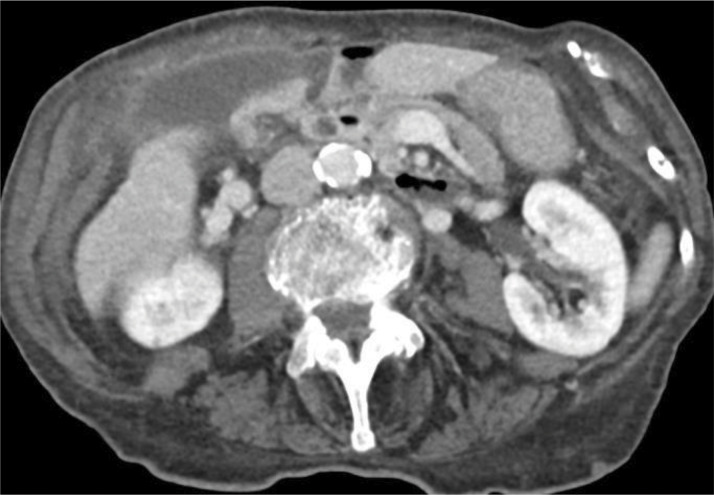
Axial image of a CT scan during the portal phase most obviously showing the “whirl sign” representing twisting of the cystic pedicle and “beak sign” at the gallbladder neck.
